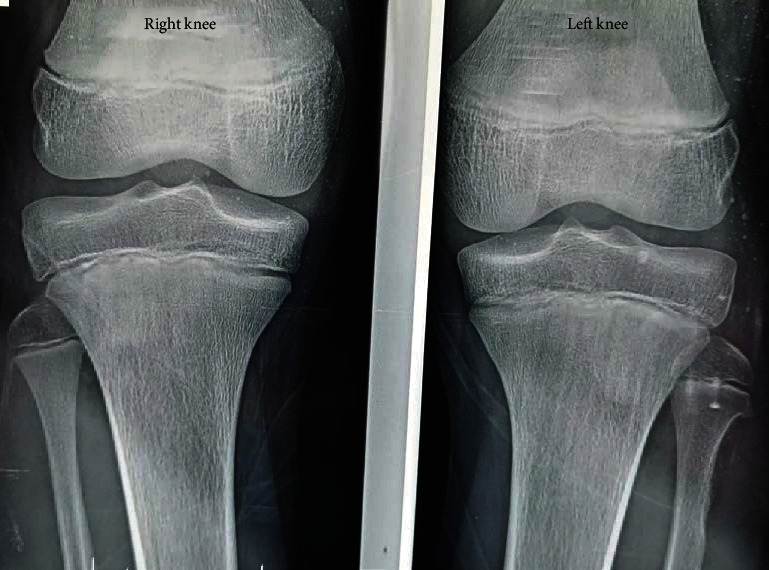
Plain X-rays of both knees directly postoperatively.
